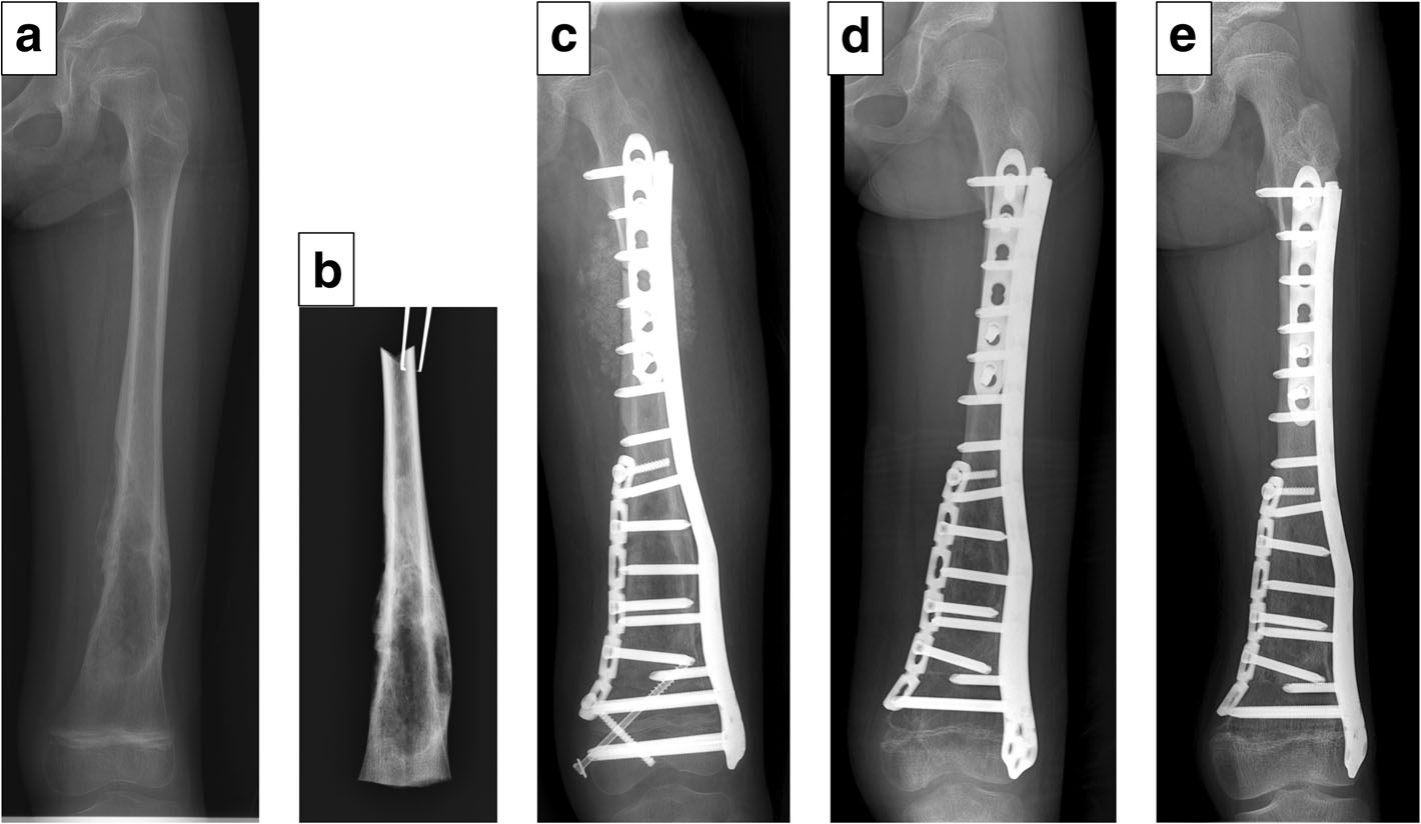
Patient No. 5. A 6-year-old girl with osteosarcoma of the left distal femur (transmetaphyseal excision). Preoperative plain radiograph (epiphysis width: 53.2 mm) (a). A radiograph of resected tumor-bearing segment of femur after transmetaphyseal excision (b). A radiograph after the reconstruction using a tumor-bearing frozen autograft stabilized with triple-locking plates and a cancellous allograft (aLDFA: 80°) (c). A radiograph after 18 months. Screws in the epiphysis were removed (d). A radiograph taken 48 months. Latitudinal and longitudinal growth were observed (aLDFA: 83°, epiphysis width: 63.4 mm) (e)
